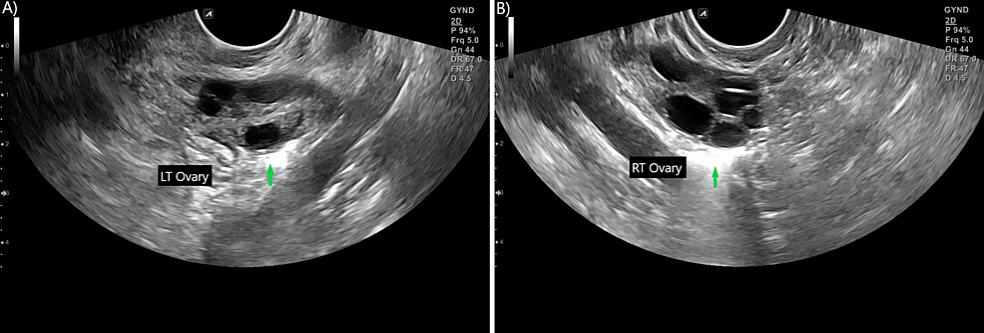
HyFoSy assessment via 2D and 3D (not shown) ultrasonography A: Left tube patent, arrow points at contrast spillage into peritoneum, B: Right tube patent, arrow points at contrast spillage into peritoneum. HyFoSy: Hysterosalpingo-foam sonography
